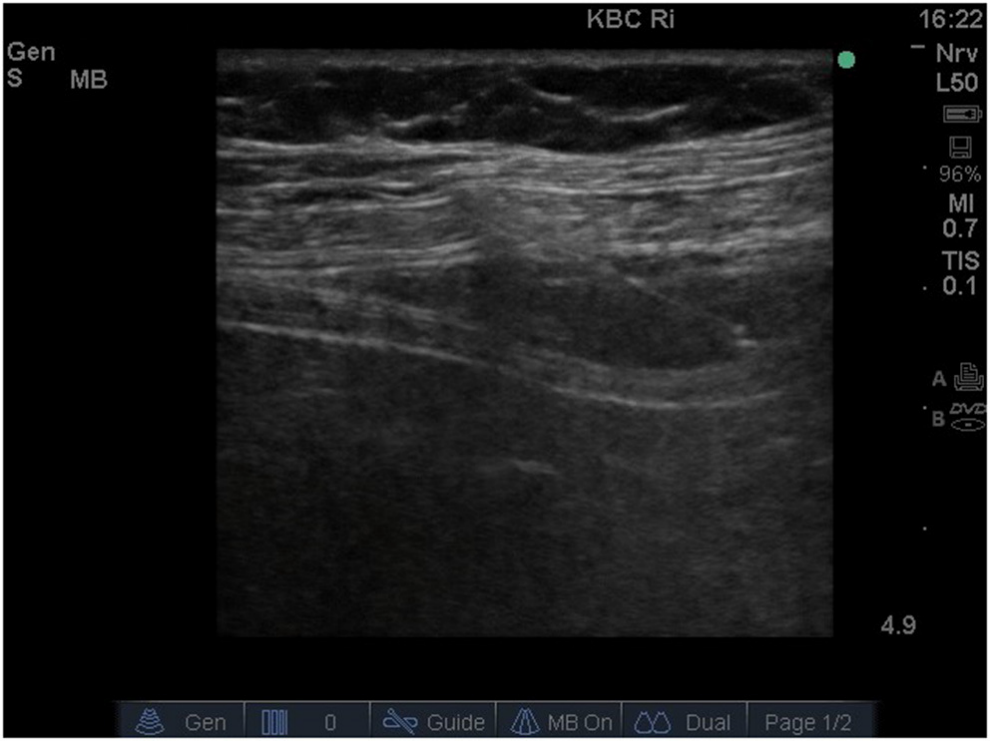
Ultrasound image demonstrating a needle placed in the space between the internal oblique and transversus abdominis muscles (transversus abdominis plane) with the injection of local anesthetic into the target area.
